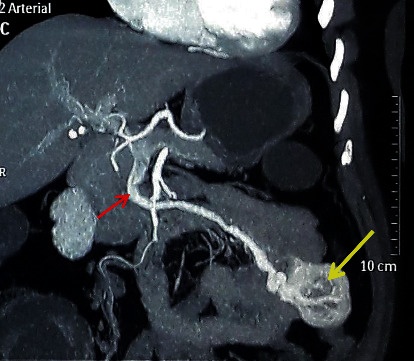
Coronal view of contrast-enhanced CT scan image arterial phase: yellow arrow shows the jejunal lesion; red arrow shows the contrast enhancement of superior mesenteric vein.
